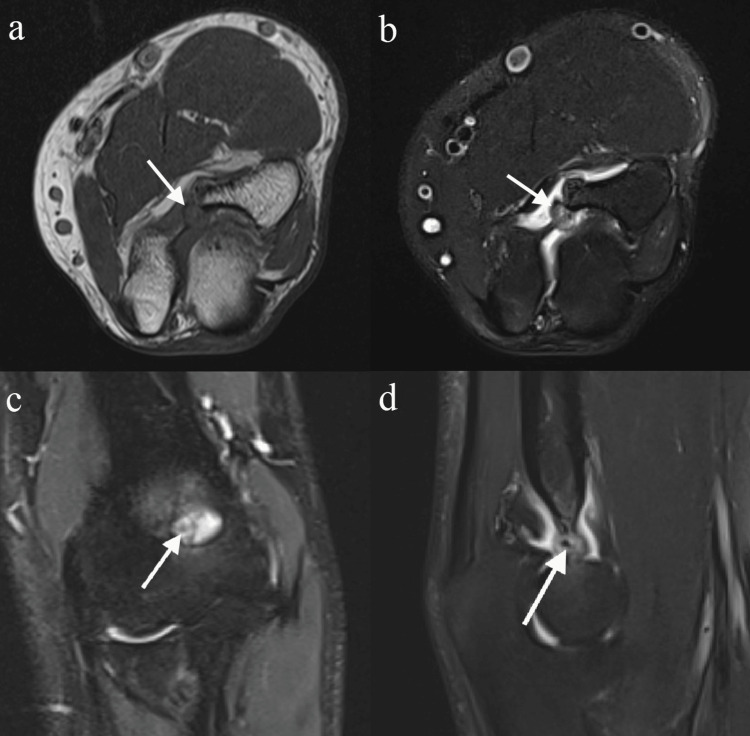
Preoperative MRI of the left elbow Axial T1 (a) and axial T2 fat-saturated (b) images demonstrate the expansile hypointense T1 and mixed hypointense/hyperintense T2 lesion arising from the olecranon fossa with thin cortical rim (arrow). Coronal T2 fat-saturated image (c) shows the central nidus (arrow) within the lesion surrounded by marrow edema. Sagittal T2 fat-saturated image (d) depicts a small hypointense nidus within the lesion (arrow) and a small effusion with some synovitis.
